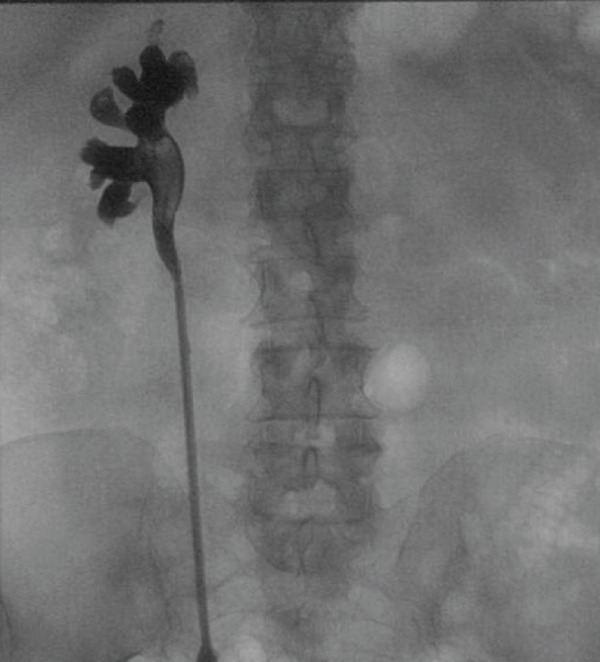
Retrograde pyelogram was obtained during definitive ureteroscopic stone removal, performed several weeks after removal of the patient′s hepatic drains. The radiograph shows no contrast extravasation, indicating complete resolution of the pyelo‐hepatic fistula. A filling defect in the ureteropelvic junction corresponds to the 2.5‐cm stone.
